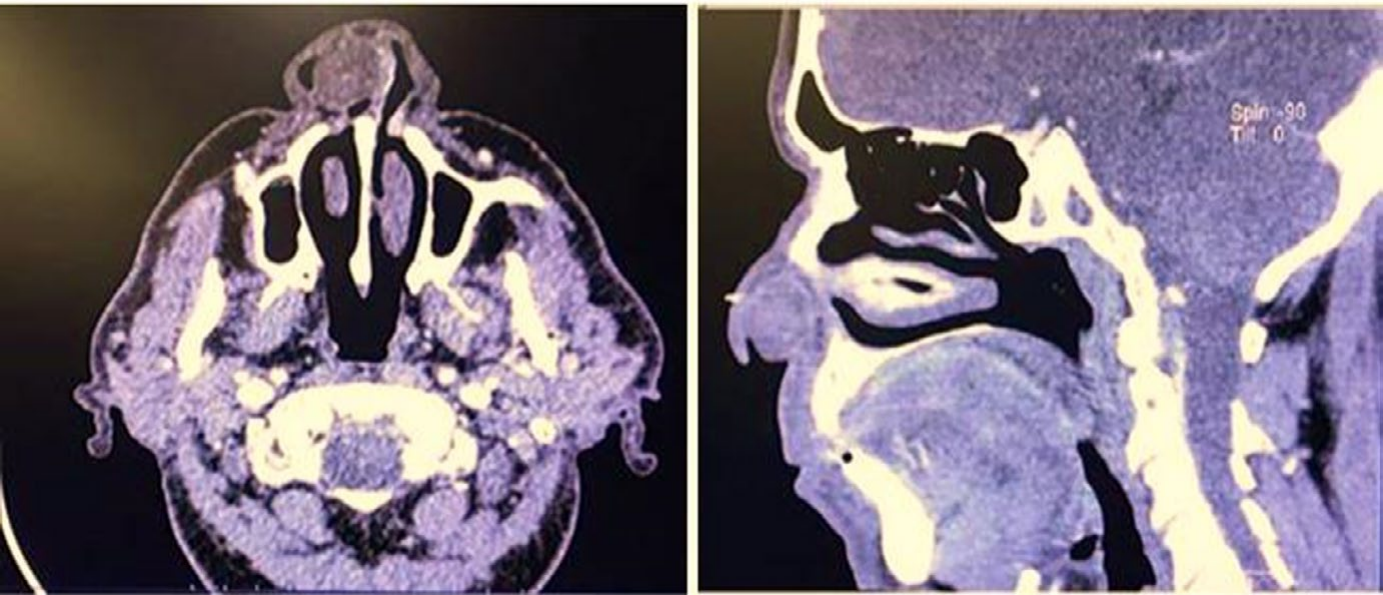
Contrast‐enhanced computed tomography showed a well‐defined hypodense lesion in the anterior part of the right nasal cavity
